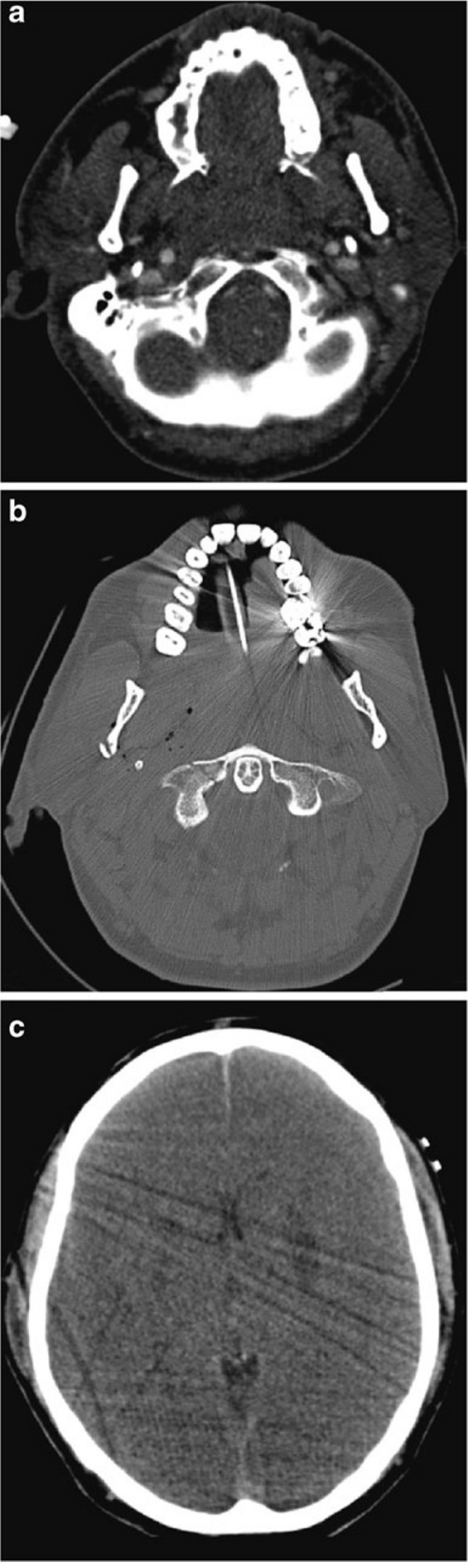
a Axial MDCTA image through the junction of the nasopharynx with the oropharynx (above the level of the endotracheal tube) in a 43-year-old man who sustained fatal perforating gunshot injury to the neck, which entered in the left zone 3 and exited through the right zone 2, having traversed the posterior oropharynx (image not shown). The pharyngeal airway at the level shown has been completely obliterated by haematoma and oedema. Pharyngeal projectile penetrating injuries, in the absence of major vascular injury, are frequently fatal due to airway compromise from haematoma, oedematous swelling and haemorrhagic debris. b Axial CT image of the same patient as in 8a on bone-window settings through the superior oropharynx (slightly inferior to the level in a). There is fracture disruption of the right lateral mass of the atlas (C1) at the junction with the anterior arch as well as fracture of the posterior aspect of the right ramus of the mandible cause by the bullet (which exited through zone 2 on this side). Soft tissue emphysema and swelling is evident in the right prevertebral, parapahryngeal and masticator spaces. The endotracheal tube is demonstrated. c Non-contrast CT brain of the same patient as in a and b performed 2 days later, demonstrating severe generalised hypoxic ischaemic encephalopathy with symmetrical subacute low attenuation change identified in the basal ganglia at this level as well as evidence of significant cerebral oedema and swelling. Brain stem death was subsequently confirmed and intensive care support withdrawn
